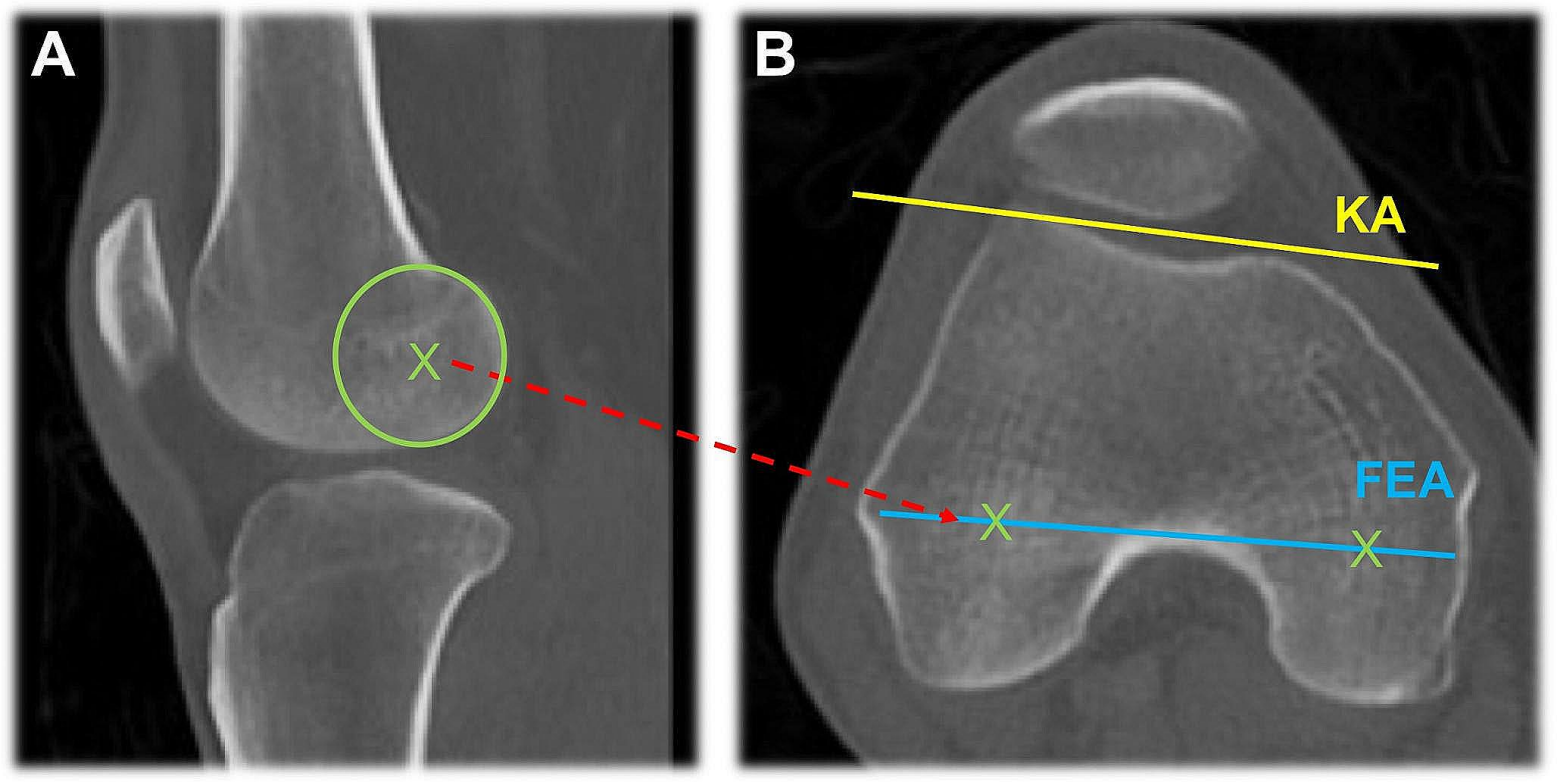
CT imaging of the knee. (A) Determination of the posterior centers of rotation marked with green “X” and (B) transfer to the axial section plane. X = posterior centers of rotation, KA = K-wire axis and FEA = flexion-extension axis. Areas refer to the bony structures
